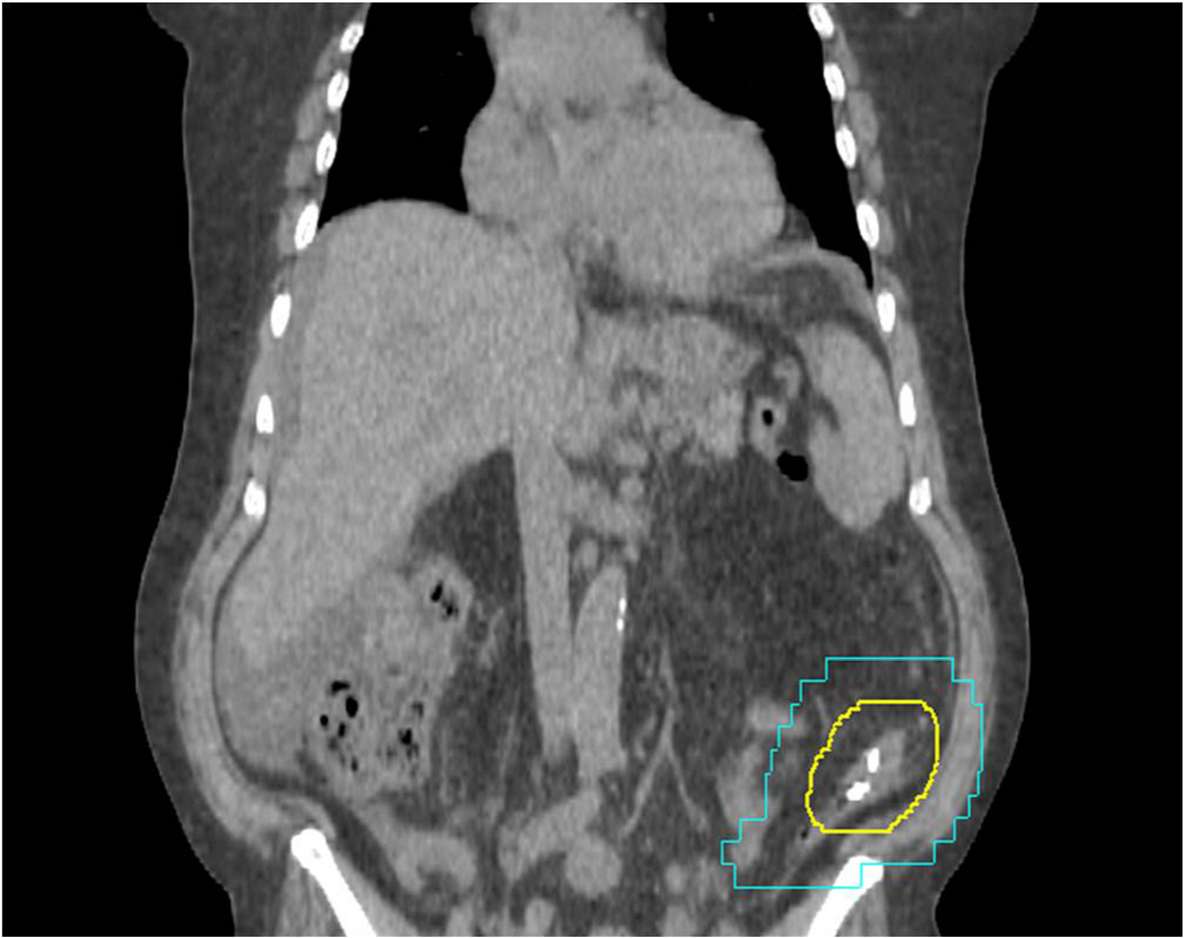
Computed tomography of palliative radiotherapy plan with clips placed. Representative coronal CT image of the CTV (yellow) and radiation field edges (blue). Three clips were placed within the tumour and at the distal and proximal extent. The PTV volume was decreased from 393.8 cm^3^ to 251.5 cm^3^. The V15 for small bowel loops was reduced from 328.0 cm^3^ to 176.0 cm^3^.
